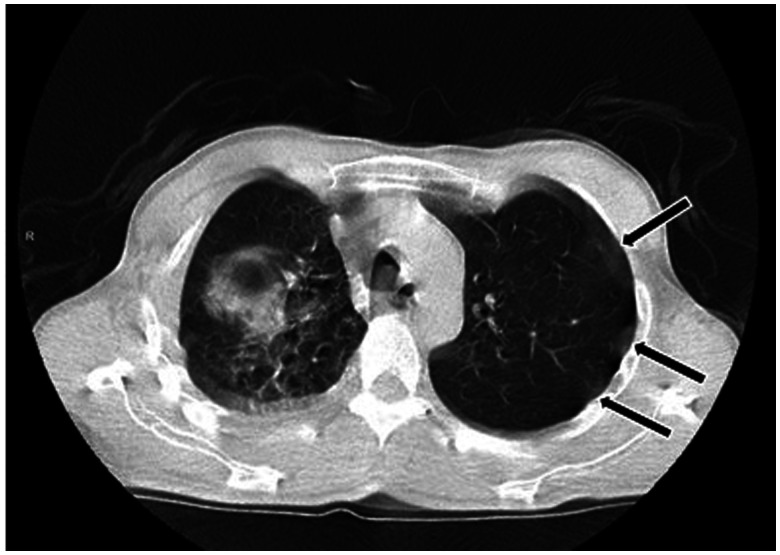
Axial reformatted unenhanced thorax computed tomography image demonstrates subpleural ground-glass opacities (arrows) in the bilateral lung.
